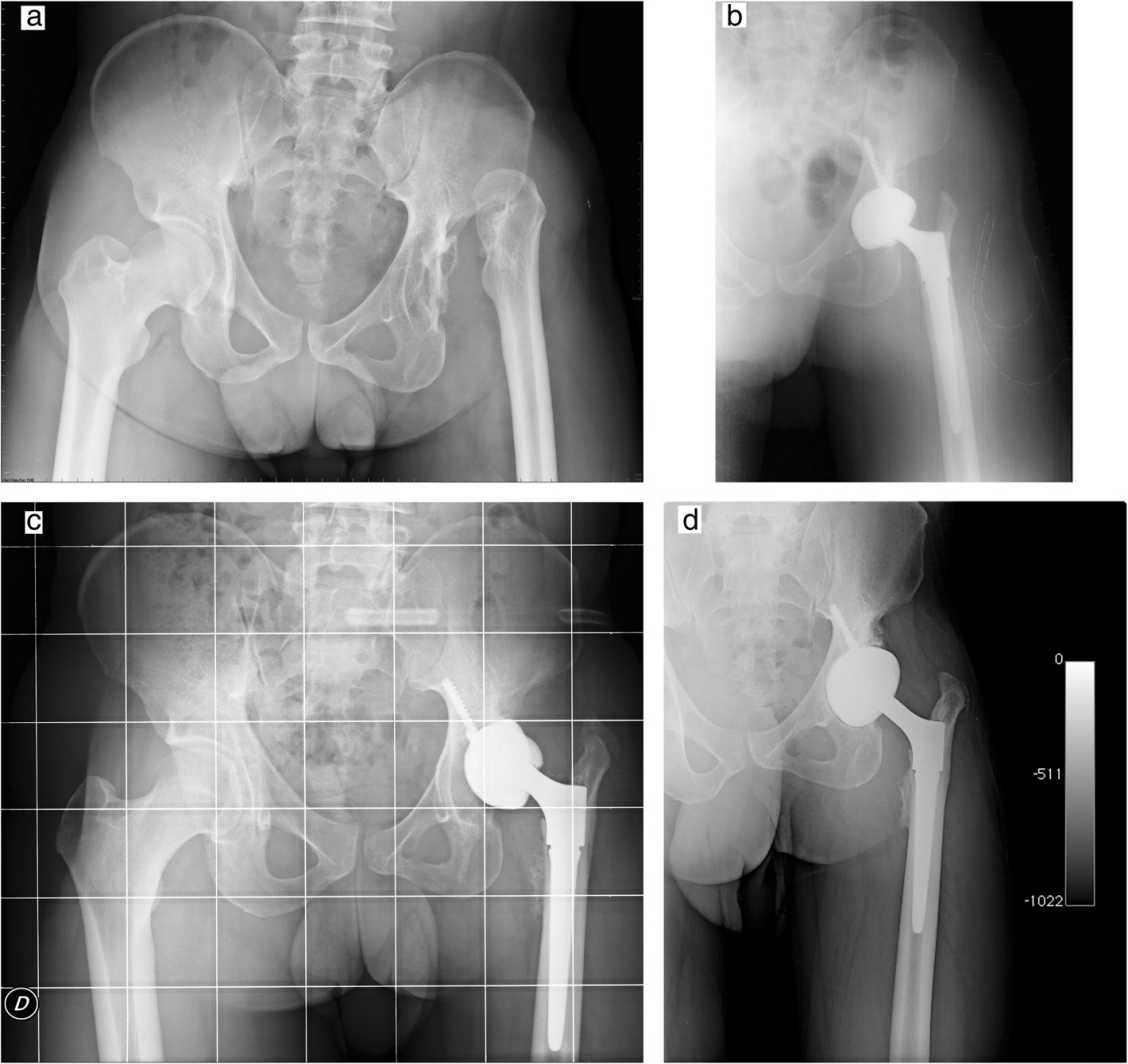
Case of cup loosening: a pre-operative X-ray (DDH Crowe 4), b post-operative radiograph, c loosening of the cup, d X-ray at 4-year follow-up, after revision with primary DELTA-TT cup
